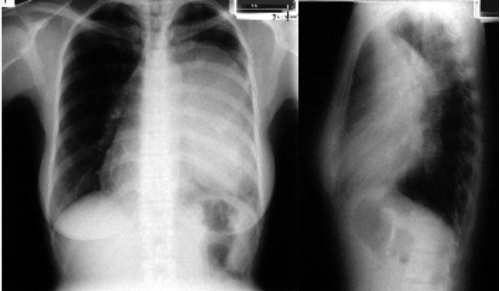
Preoperative chest X-ray showing total atelectasis of the left lung and deviation of the cardiac silhouette to the right.
